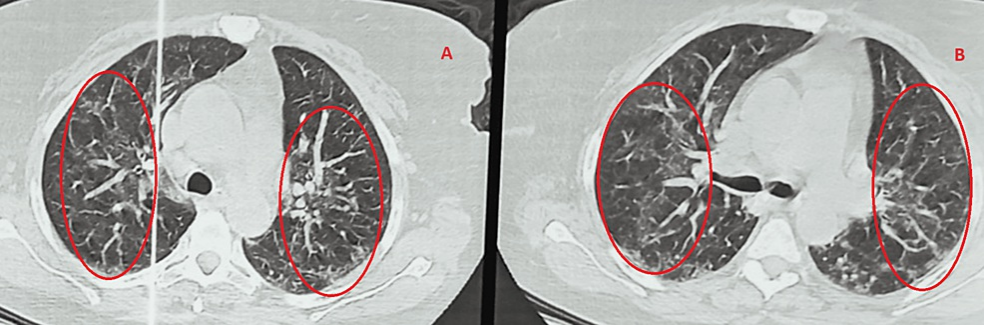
Chest CT scan performed in 2021 showing a bilateral frosted-glass image and septal thickening realizing the crazy paving appearance during her suspected COVID infection.
